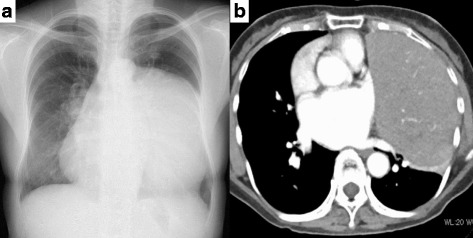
a Chest X-ray shows a very large mass in the left middle and lower lung fields. b Chest computed tomography shows a tumor (16 × 10 × 7 cm) in direct contact with a large area of the chest wall and diaphragm, and pressing on the heart and left upper pulmonary lobe
